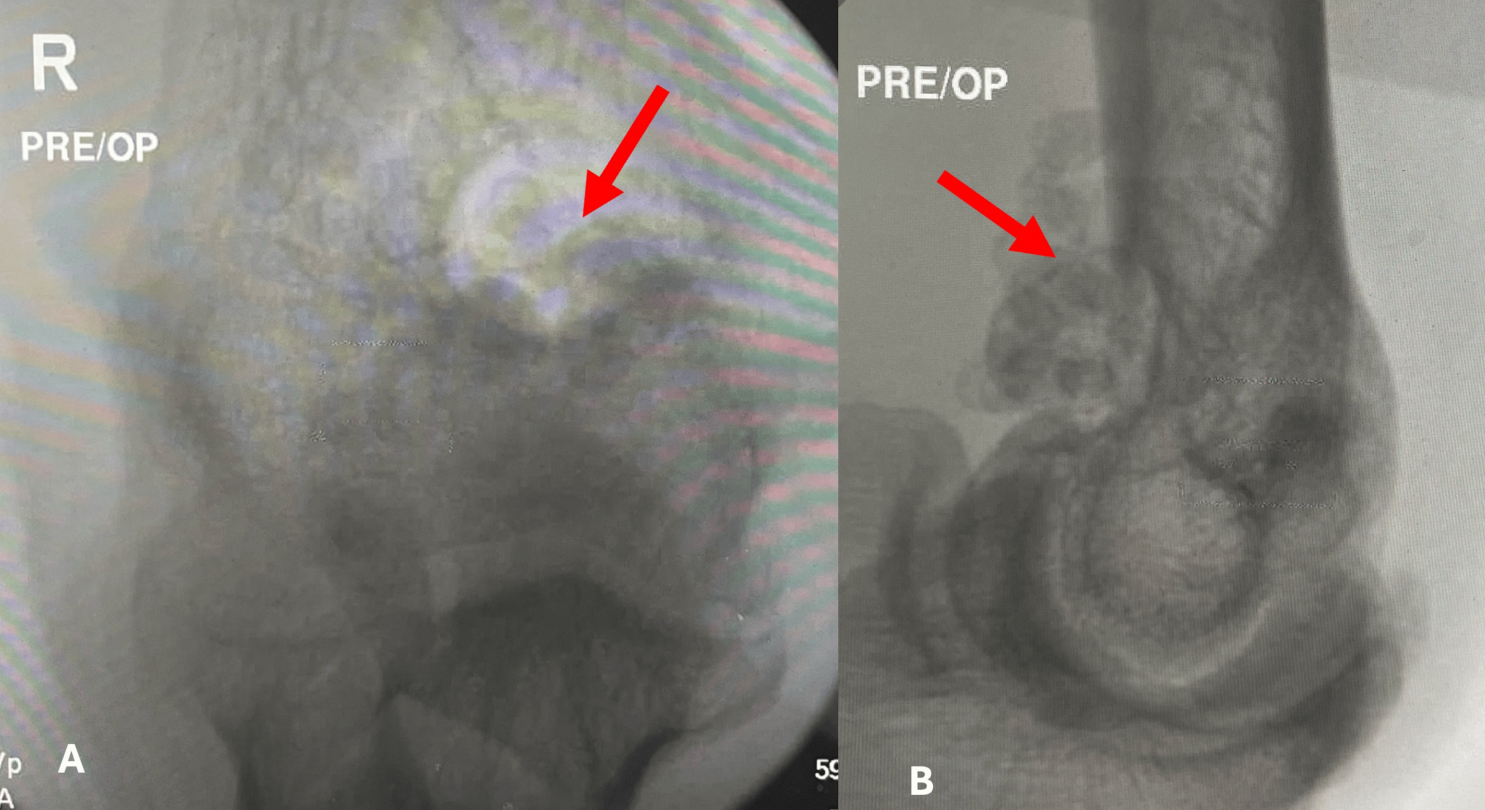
Pre-operative radiographs of the right elbow Pre-operative (A) anterior-posterior and (B) lateral radiographs of the right elbow demonstrating multiple loose bodies in the elbow joint (red arrows).
